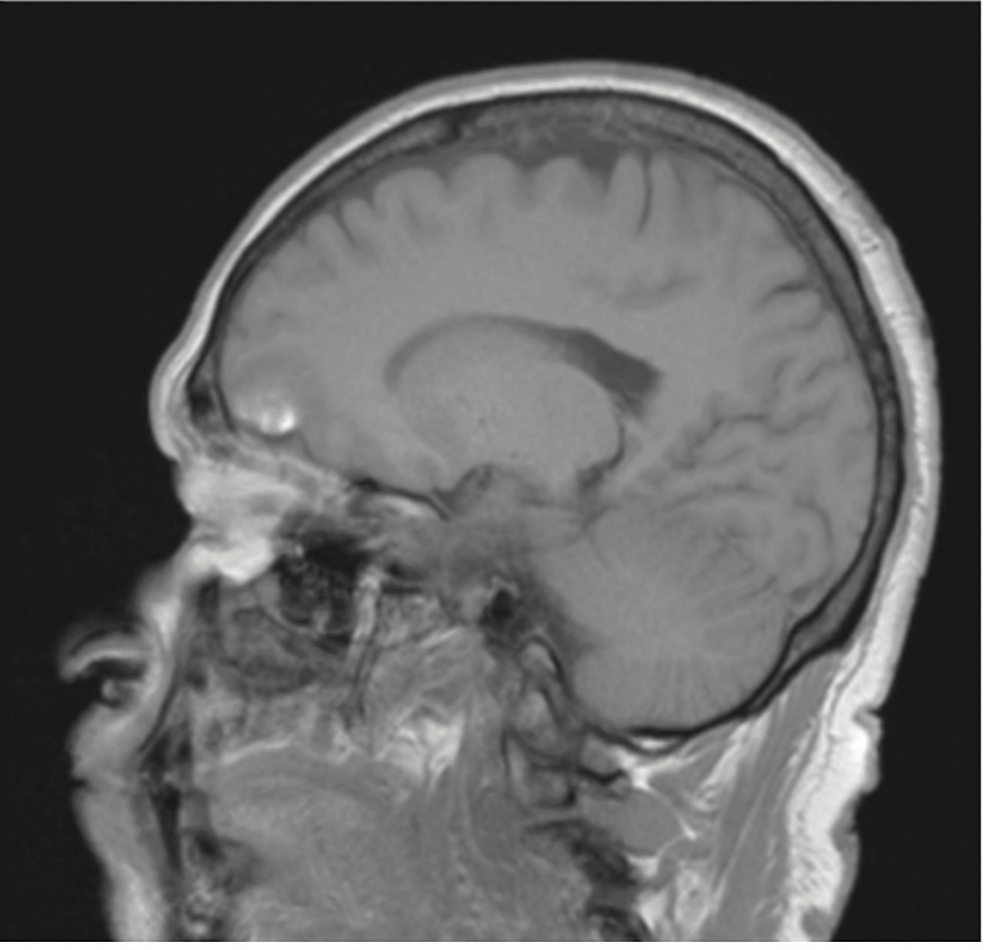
Sagittal T1 MRI Brain. Hyperintense lesion in frontal lobe suggestive of hemorrhage.
